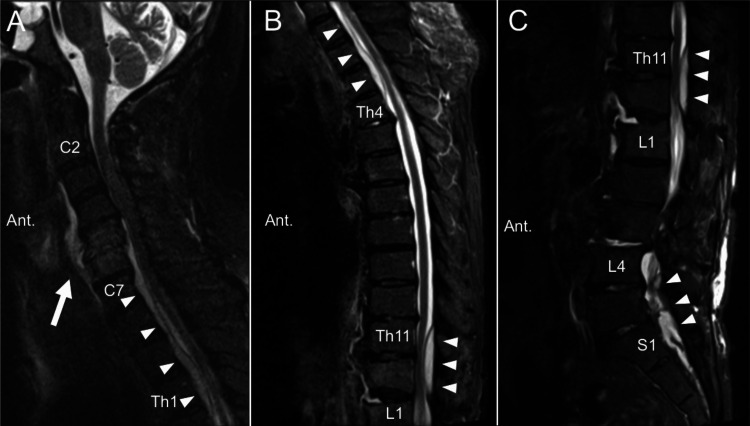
Preoperative radiological images. Sagittal whole-spine T2-weighted magnetic resonance images showing a retropharyngeal abscess (white arrow) and epidural abscess (white arrowheads). Hyperintensity of C5 and C6 vertebral bodies suggest pyogenic spondylitis. Fluid collection in the intervertebral spaces of C5/6, Th12/L1, L3/4, L4/5, and L5/S1 appear to be spondylodiscitis (A: cervicothoracic regions, B: thoracic regions, C: thoracic-lumbar-sacral regions).
